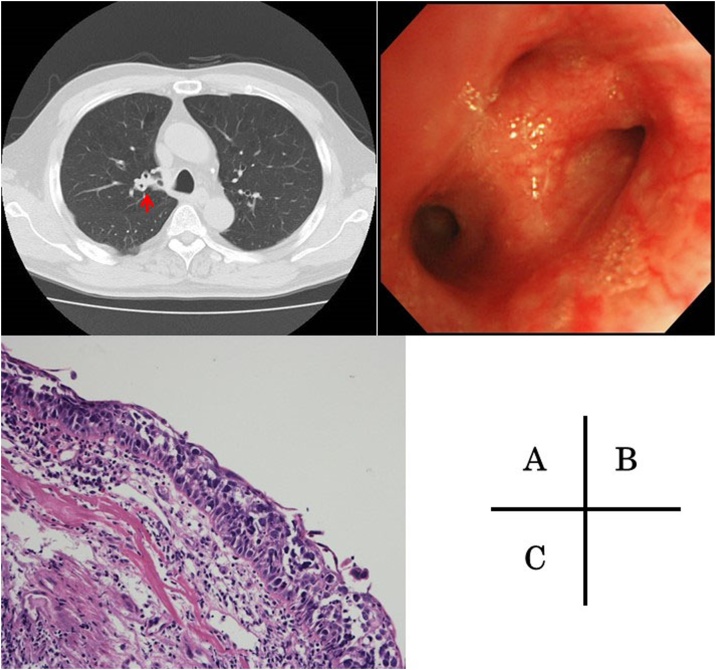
Clinical and pathological findings of the tumor recurrence. *A*, Computed tomography revealed a thickened right upper bronchus. *B, C*, Bronchoscopy revealed that the epithelium of the right upper bronchus was reddish and thick. Transbronchial biopsy yielded a pathological diagnosis of a large cell neuroendocrine carcinoma with bronchial intraepithelial recurrence.
